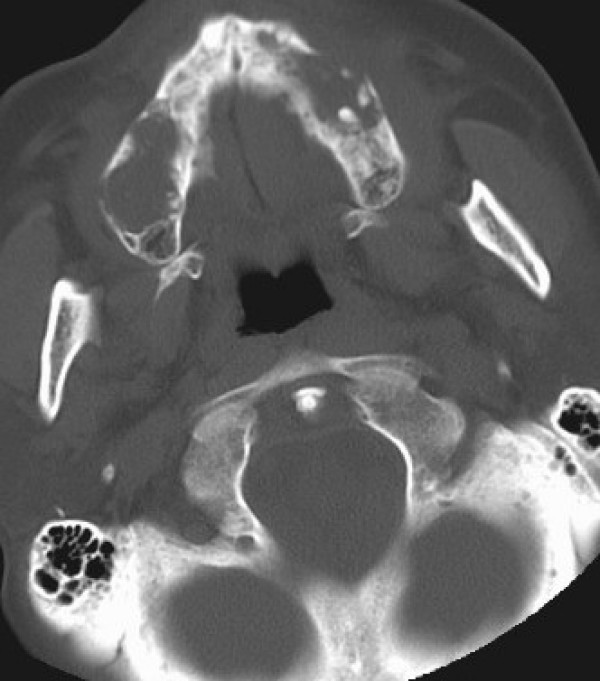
Facial computed tomography scan (Axial view, bone windows): Multiple odontogenic keratocysts are present in the upper mandible.
